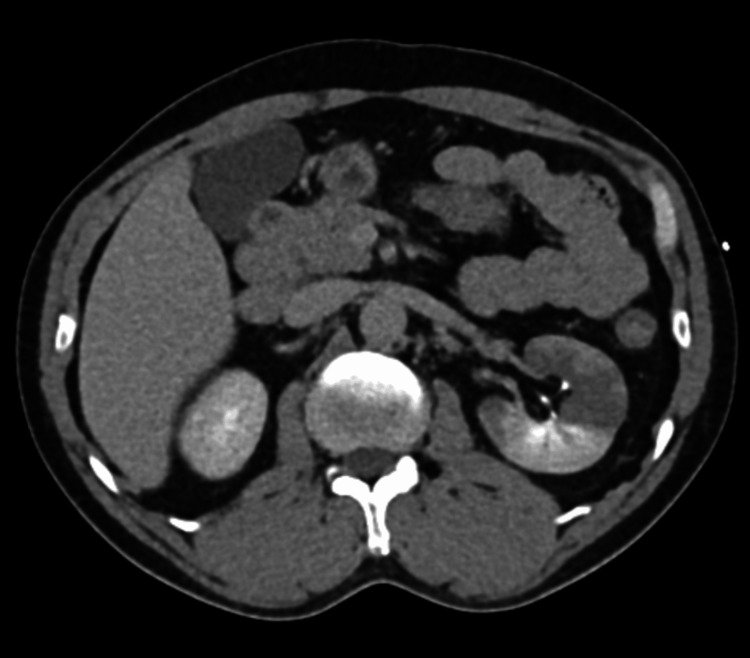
CT (axial view) showing acute infarction of the superior pole of the kidney with wedge-shaped perfusion defect secondary to thrombosis of variant superior polar artery
